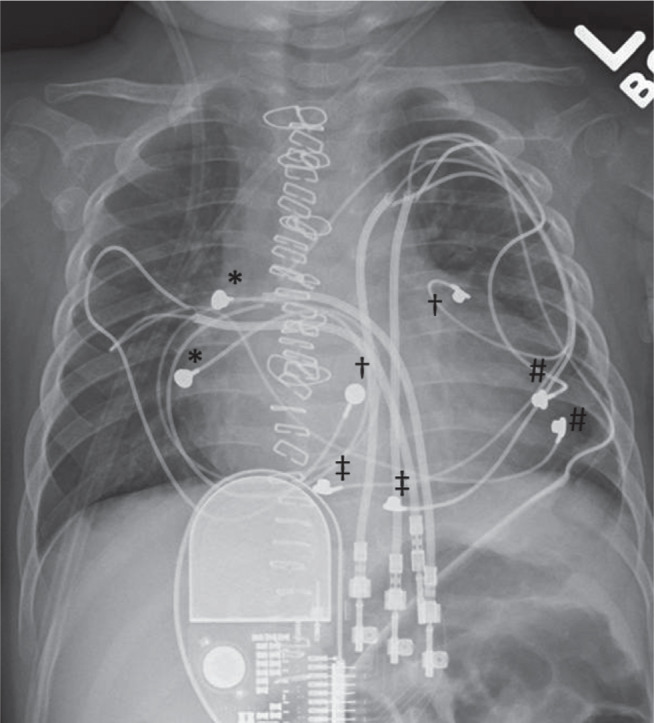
Chest radiograph demonstrating the epicardial pacing system. ^*^Bipolar atrial lead (chronic lead); ^#^bipolar LV apical lead (chronic lead); ^†^bipolar RV lead; ^‡^bipolar LV basolateral lead.
